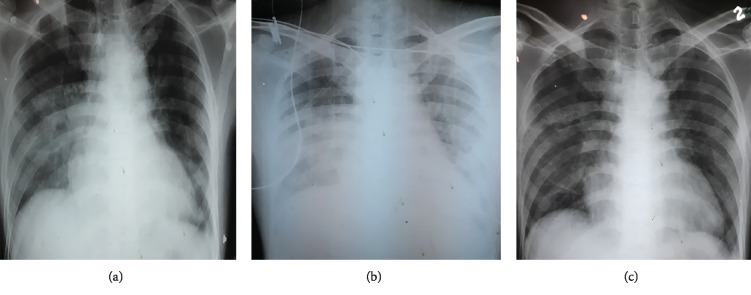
(a) Chest radiograph showing bilateral middle and lower zone alveolar shadows more on the right lung with obliterated left costophrenic angle and (b) chest radiograph revealing more prominent diffuse bilateral opacities and (c) resolution of shadows after steroid treatment.
